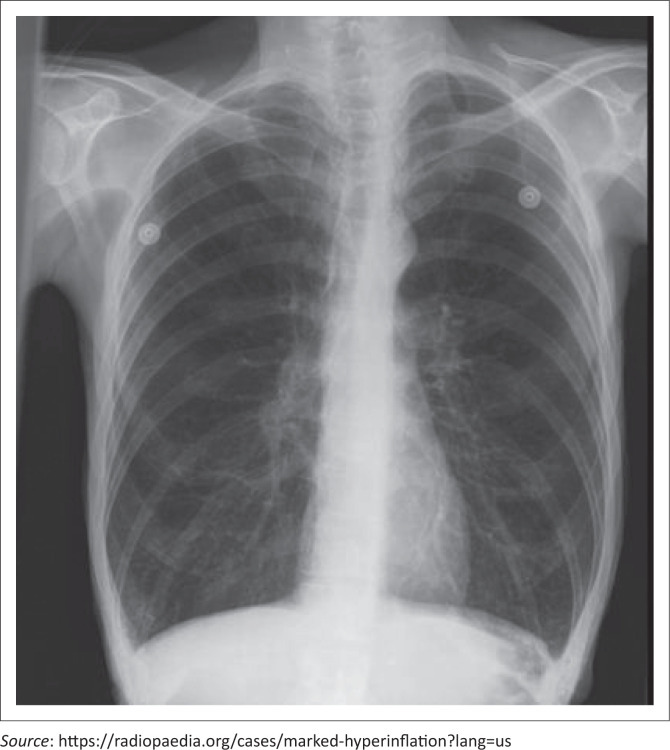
Chest X-ray for objectively structured clinical examination station (main finding: only hyperinflated).
